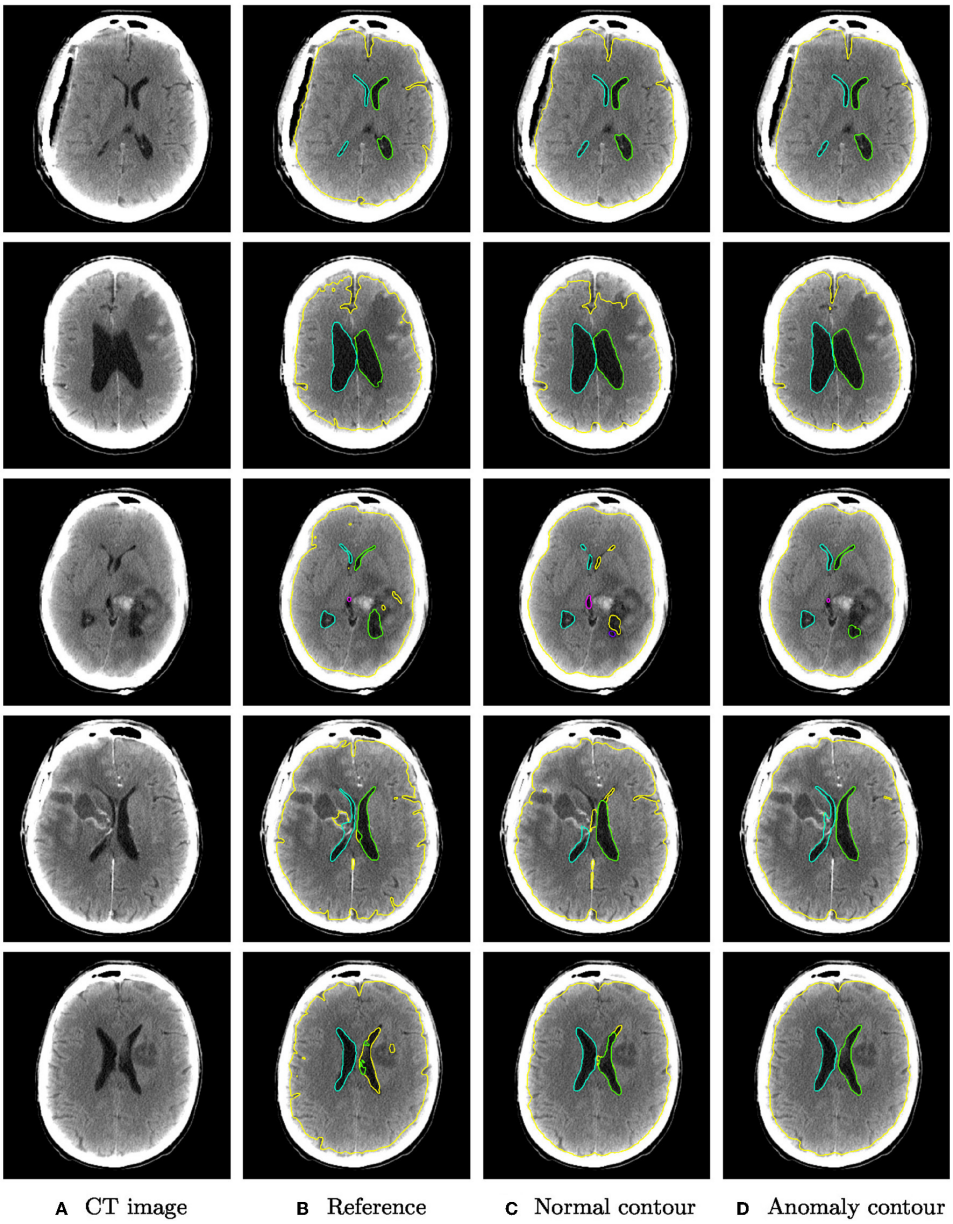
Qualitative segmentation examples from the glioma dataset of parenchyma including tumors (yellow) and ventricles (left ventricle: green, right ventricle: cyan, third ventricle: magenta, fourth ventricle: purple). Each row shows from left to right (A) the original CT slices, (B) the reference segmentation, (C) the segmentation from the algorithm trained on normal cases only, and (D) the segmentation from the algorithm trained on normal cases and hemorrhage cases.
